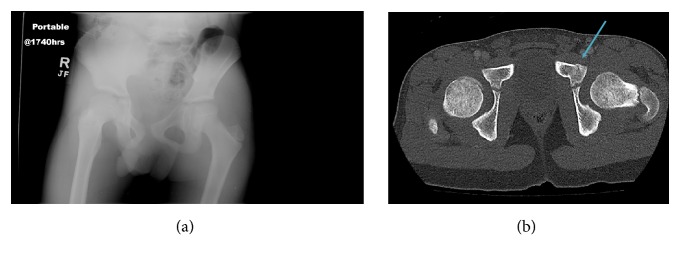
A portable radiograph of the pelvis (a) was performed on a 7-year-old male who was the victim of an automobile versus pedestrian trauma. No fracture or dislocation is seen on the radiograph. Twenty-nine minutes later, a contrast-enhanced CT of the abdomen and pelvis (b) was performed on the same patient demonstrating a nondisplaced left superior pubic ramus fracture (arrow).
